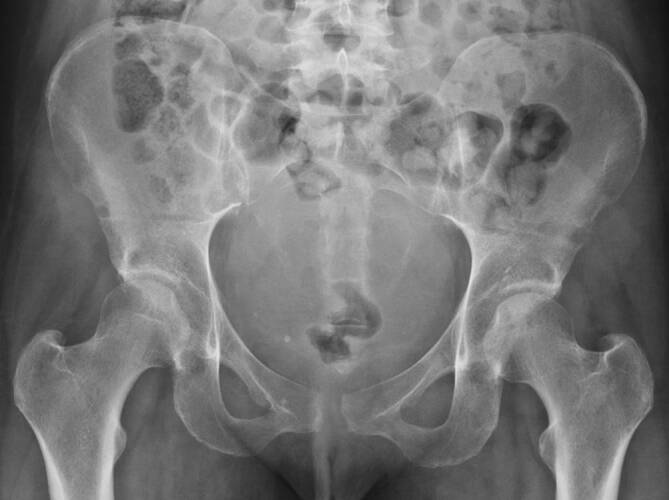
Nach 18 Wochen berichtete die Patientin wieder annähernd beschwerdefrei und uneingeschränkt ihrem Alltag und der Kinderfürsorge nachgehen zu können. In der letzten klinischen Untersuchung 6 Monate nach dem Trauma bestanden ein flüssiges Gangbild, freier Bewegungsumfang in beiden Hüftgelenken und annähernde Schmerzfreiheit. Der Kraftgrad bei der Hüftbeugung betrug 5/5. Die sonographische Kontrolle zeigte eine konstante Weite des Symphysenspalts von 12 mm (Abb. 6) ohne sekundäre Dislokation. Erstmals äußerte die Patientin jedoch sensible Defizite mit Hypästhesie im Bereich des ventralen Oberschenkels und insbesondere in der linken äußeren Labia, welche im Verlauf nur dezent rückläufig seien. Retrospektiv kann nicht eruiert werden, ob diese Beschwerden direkt post partum oder postoperativ nach Anlage des Beckenfixateurs aufgetreten waren.
